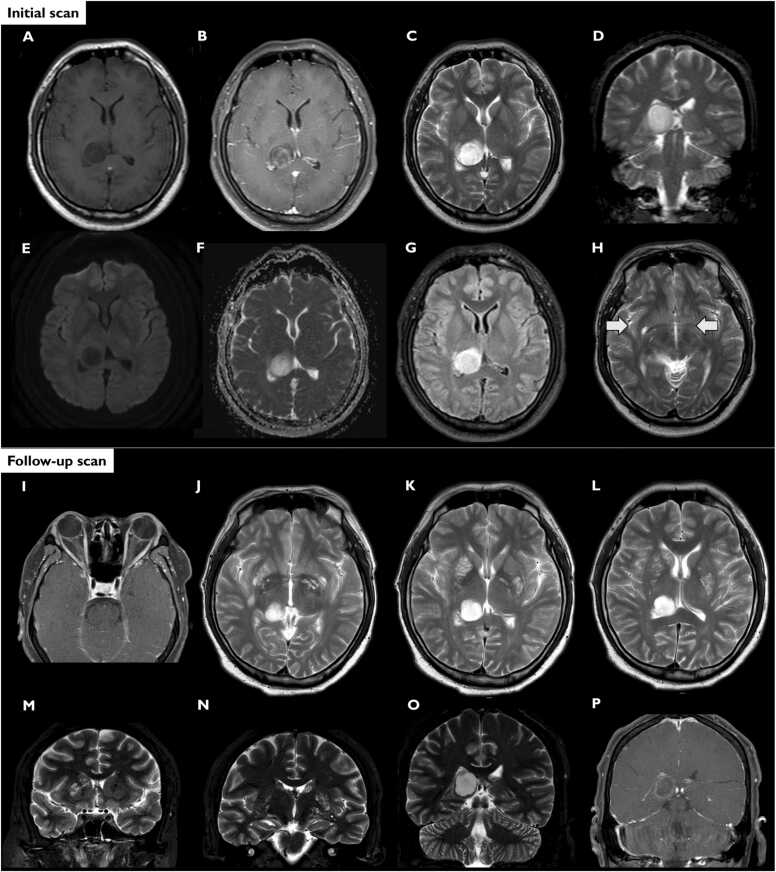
Initial and follow-up MRI brain. T1-weighted (A), T1-weighted with gadolinium (B), T2-weighted (C, D, H), diffusion-weighted images (DWI) (E), apparent diffusion coefficient (ADC) (F), and T2-fluid-attenuated inversion recovery (FLAIR) (G).
